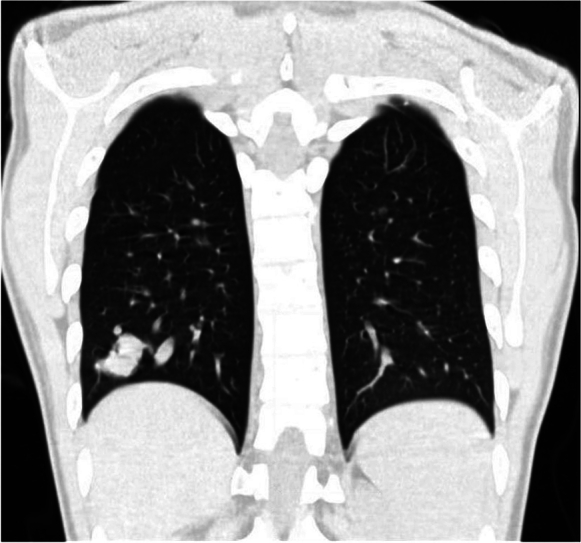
CT chest scan, coronal view: right lower lobe pulmonary arteriovenous malformation measuring 2.6 cm.
